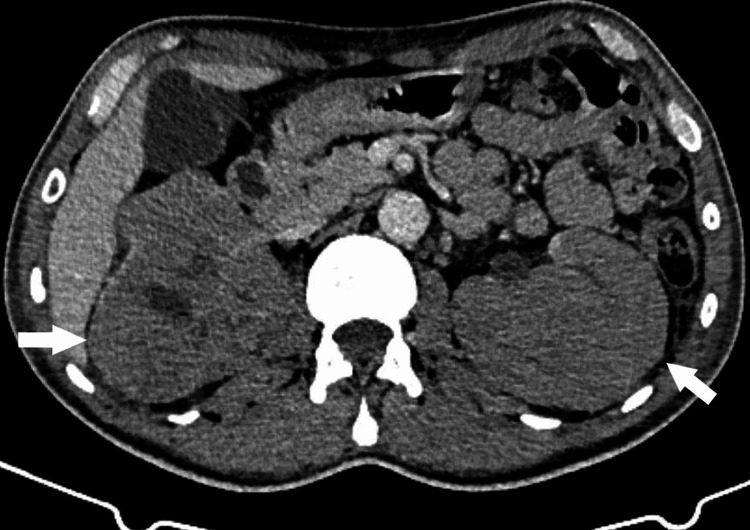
Axial abdominal CT scan showing bilateral nephromegaly with hypodense parenchymal infiltrates, without extracapsular extension, compatible with renal lymphoma.
